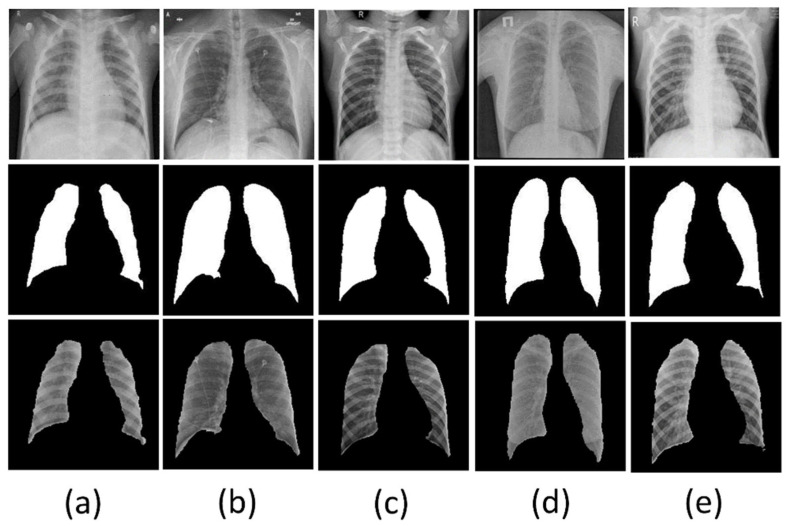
Example of images from classes (a): Bacterial Pneumonia, (b): COVID-19, (c): Normal, (d): Tuberculosis, (e): Viral Pneumonia; top row: original chest X-ray images, middle row: UNet generated corresponding masks, bottom row: final segmented lung images.
